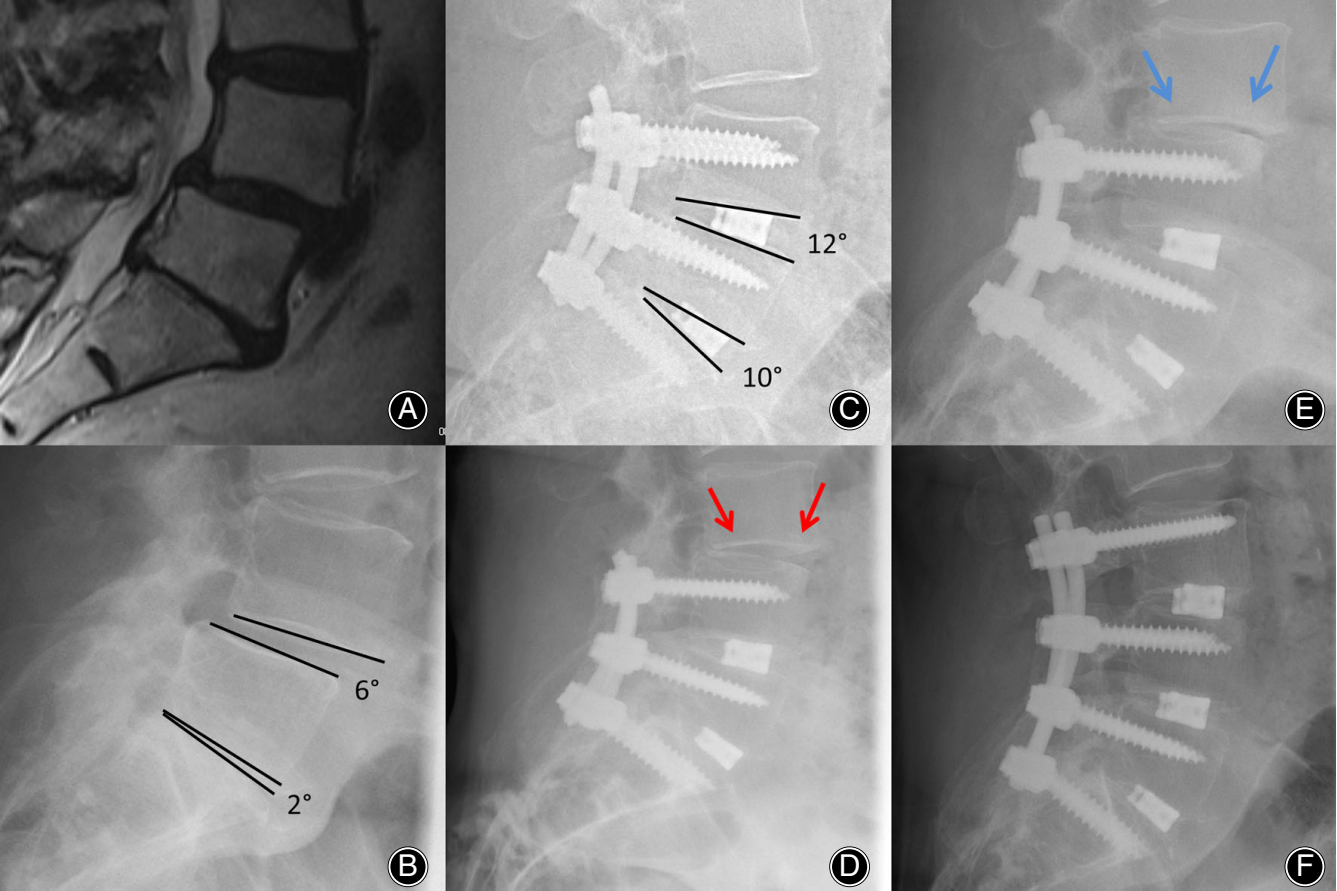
(A) Preoperative MRI scan of a 66‐year old woman with spondylolisthesis (Meyerding degree I) L4/5 and osteochondrosis L5/S1 (B) Preoperative lateral X‐ray shows a segmental lordosis L 4/5 of 6 ° and L5/S1 of 2°. (C) The follow‐up 3 months after surgery with bi‐segmental fusion and implantation of lordotic cages shows an improved segmental lordosis of 12° and 10° (increase of 6° and 8°). (D) The X‐ray control after 2 years shows a beginning adjacent disc degeneration (red arrows), which is increasing after 3 years (blue arrows, (E)) and requiring an extension of the fusion (F).
